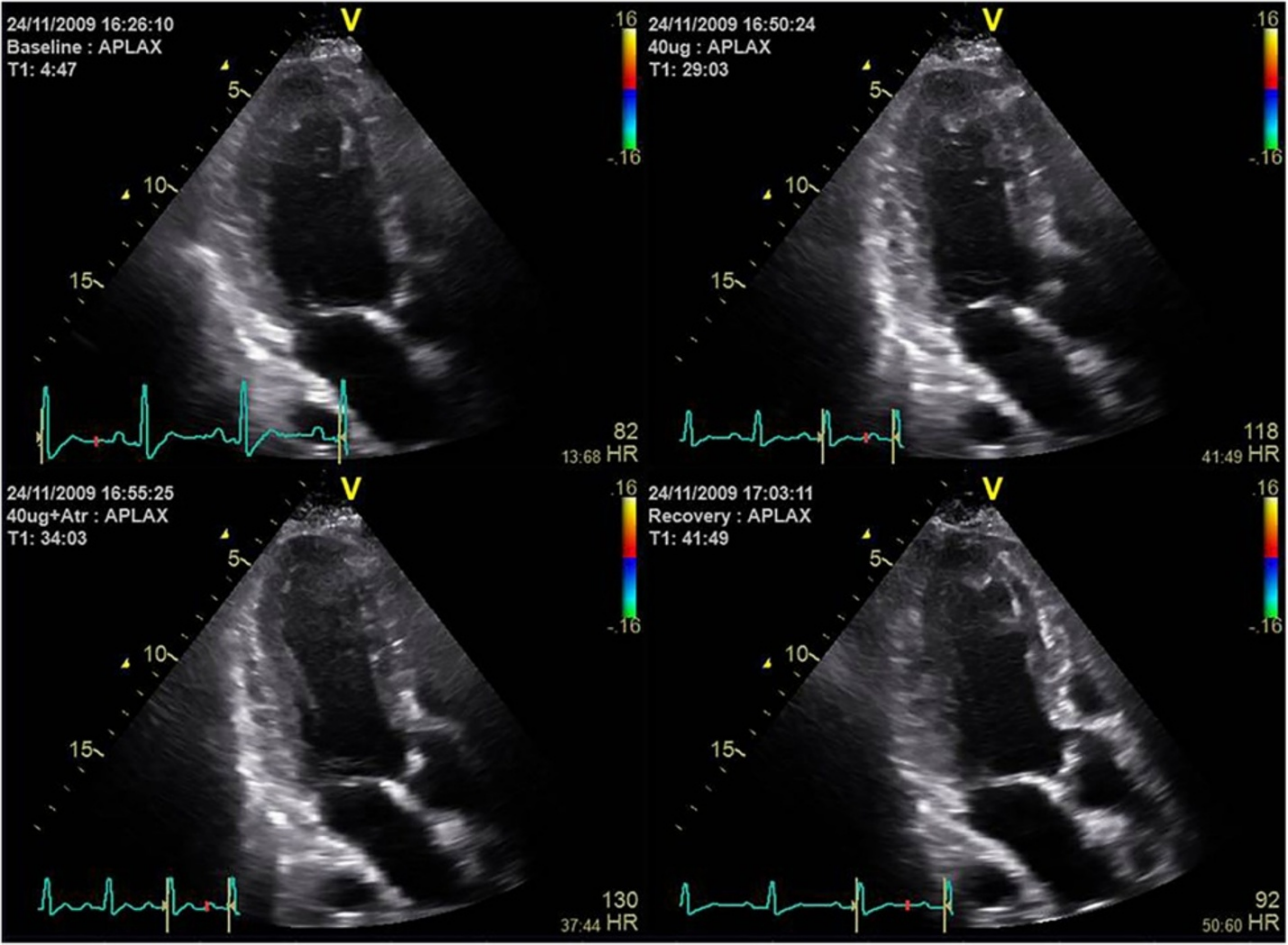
For the optimal visual assessment of wall motion in stress echocardiograms, a synchronized replay of the different stress stages of the examination in a quad-screen display is crucial. Note the colour bars in the image which indicate that colour tissue Doppler data have been acquired simultaneously which allow the quantification of myocardial function.
